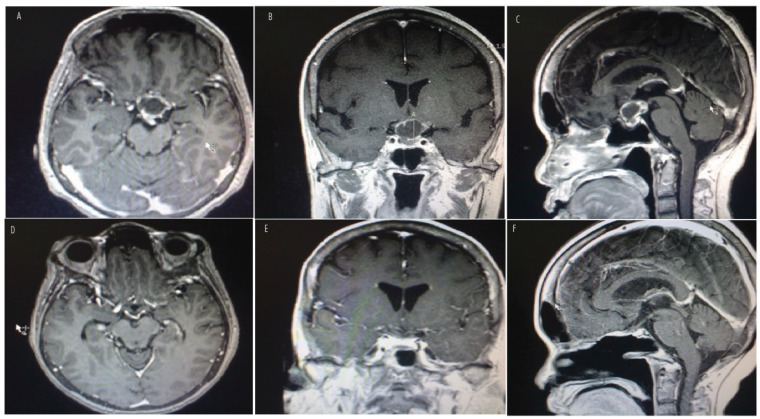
Preoperative axial, coronal, and sagittal MR images with contrast (top row, A–C) of a 12-year-old boy presenting with peripheral vision loss and diabetes insipidus. Physical examination revealed a bitemporal hemianopsia. Imaging demonstrated a predominantly multicystic and solid suprasellar mass extending inferiorly into the subdiaphragmatic space, causing a chiasmatic mass effect that was partially calcified on CT imaging. The patient underwent an endoscopic endonasal approach with gross total resection with vascularized nasoseptal flap for skull base dural reconstruction. Postoperative MR imaging (bottom row, D–F) revealed chiasmatic decompression and no residual tumor. His vision improved and final pathology revealed adamantinomatous craniopharyngioma.
